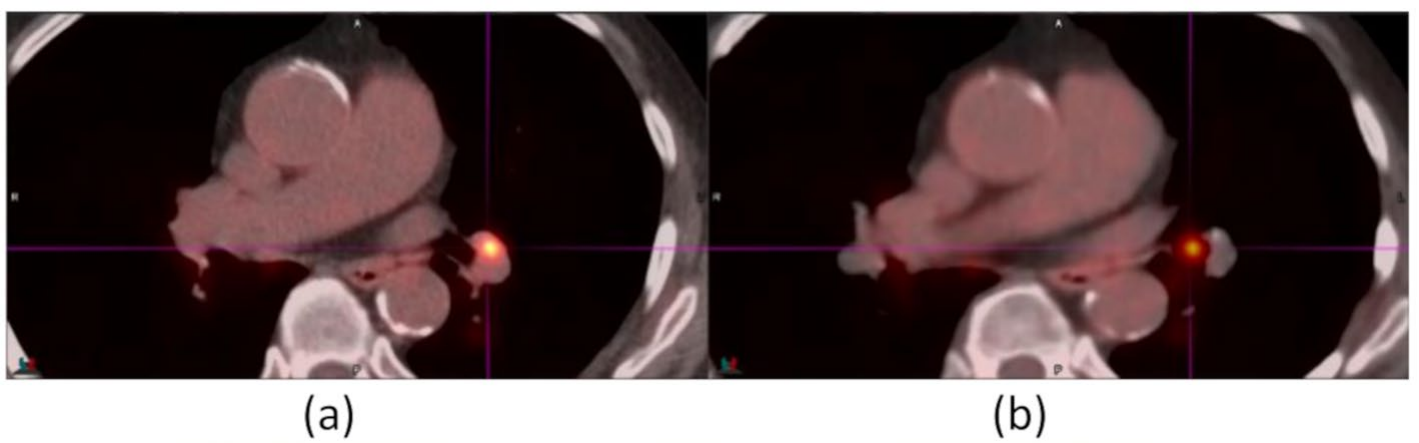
A 0.6-cm left infra-hilar lymph node was registered in baseline (a) but misregistered in AIR (b). The SUV_max_ values were 8.5 for baseline and 8.7 for AIR
